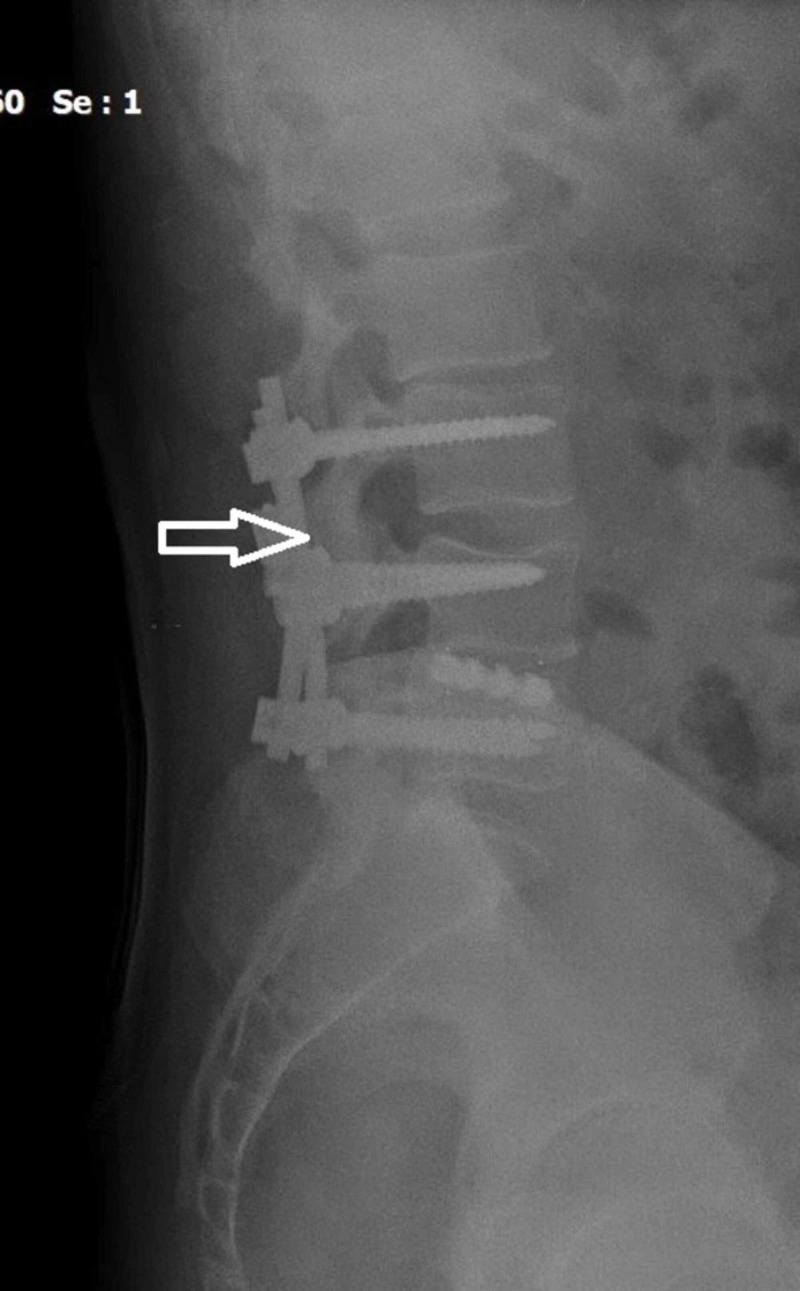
Postoperative lateral standing roentgenogram of the lumbar spine four years postoperatively. Note the completed fusion (white arrow) and excellent sagittal balance.
